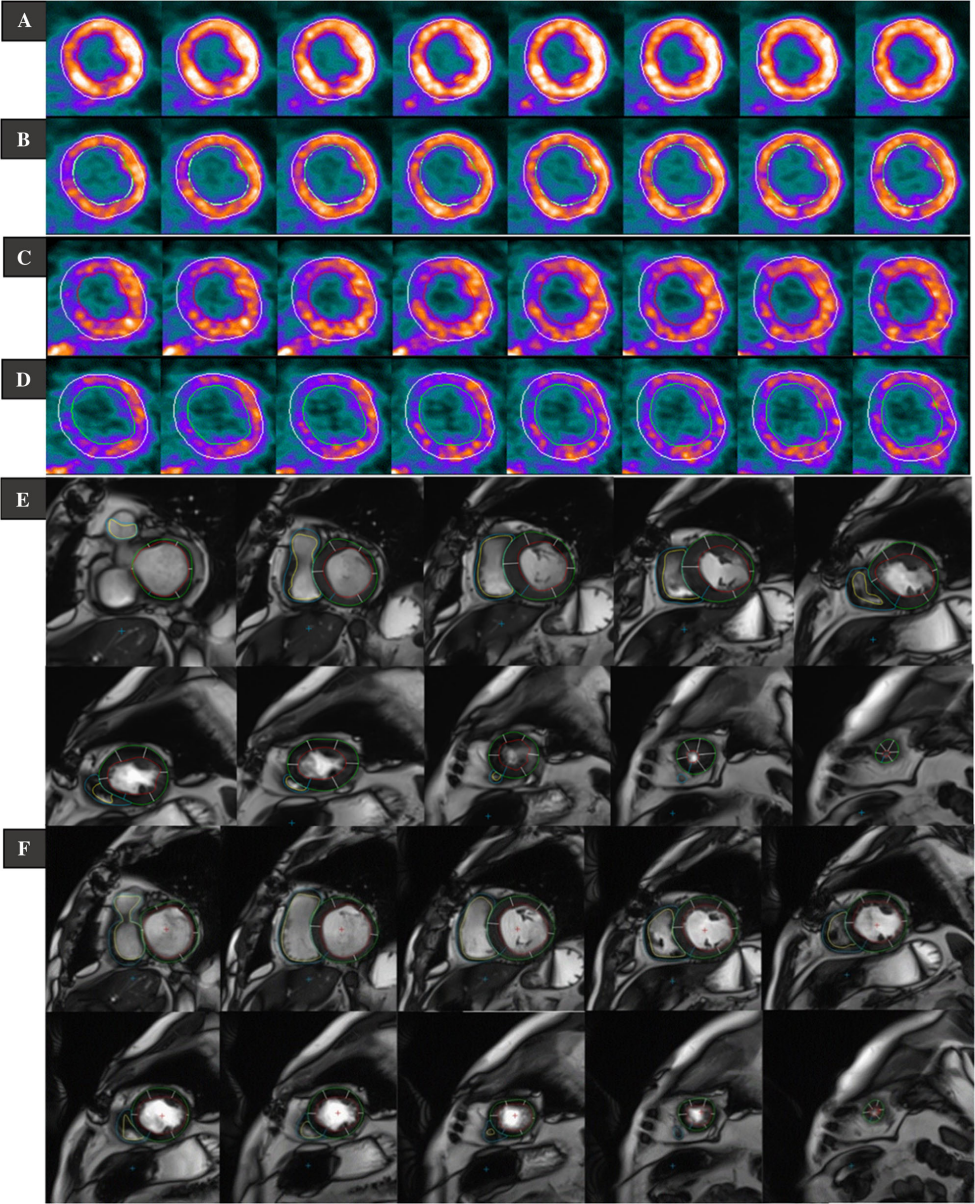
Automated delineation of the left ventricle (LV) for estimation of late-phase cardiac function of left using the software Cedars-Sinai Medical Center: (A) FDG in end systole and (B) in end diastole. (C) NH_3_ in end systole and (D) in end diastole. Manual delineation of left and right ventricle in CMR of both right ventricle (RV) and LV: (E) in end systole and (F) in end diastole
